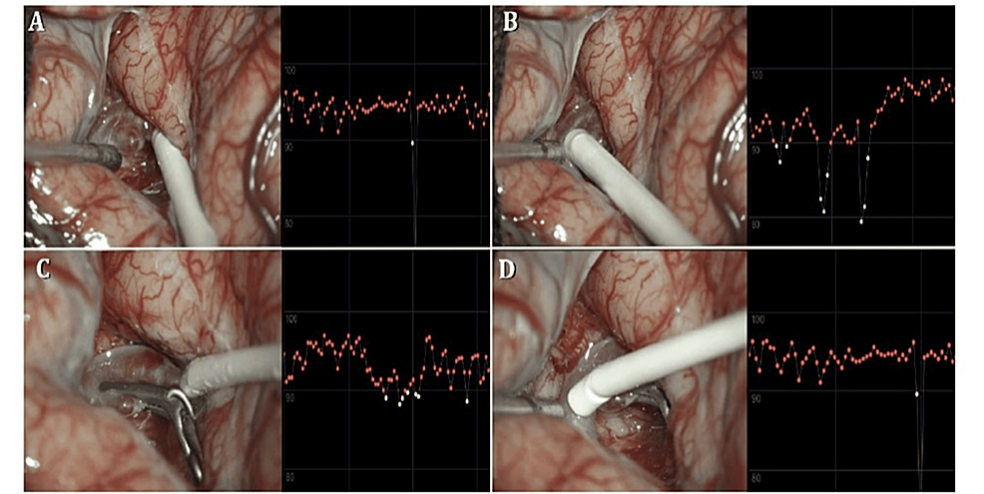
Patient two. (A) Exposes a Sylvian aneurysm at a left bifurcation. Here, the Doppler is placed on the superior or frontoparietal branch of the middle cerebral artery. The Doppler dB reading is shown on the right margin, highlighting that the superior branch has an intensity of 67 dB. (B) The inferior temporal branch averages at 63 dB. (C) After clipping, the intensity remains consistent at 67 dB. (D) Shows the average dB reading.
